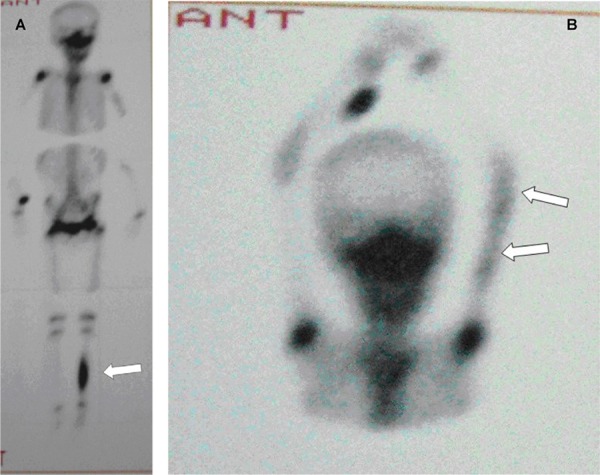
A. Bone scintigraphy three hours after i.v. injection Tc99m show active pathology in the left tibia and, B. in entire length of the left humerus.
